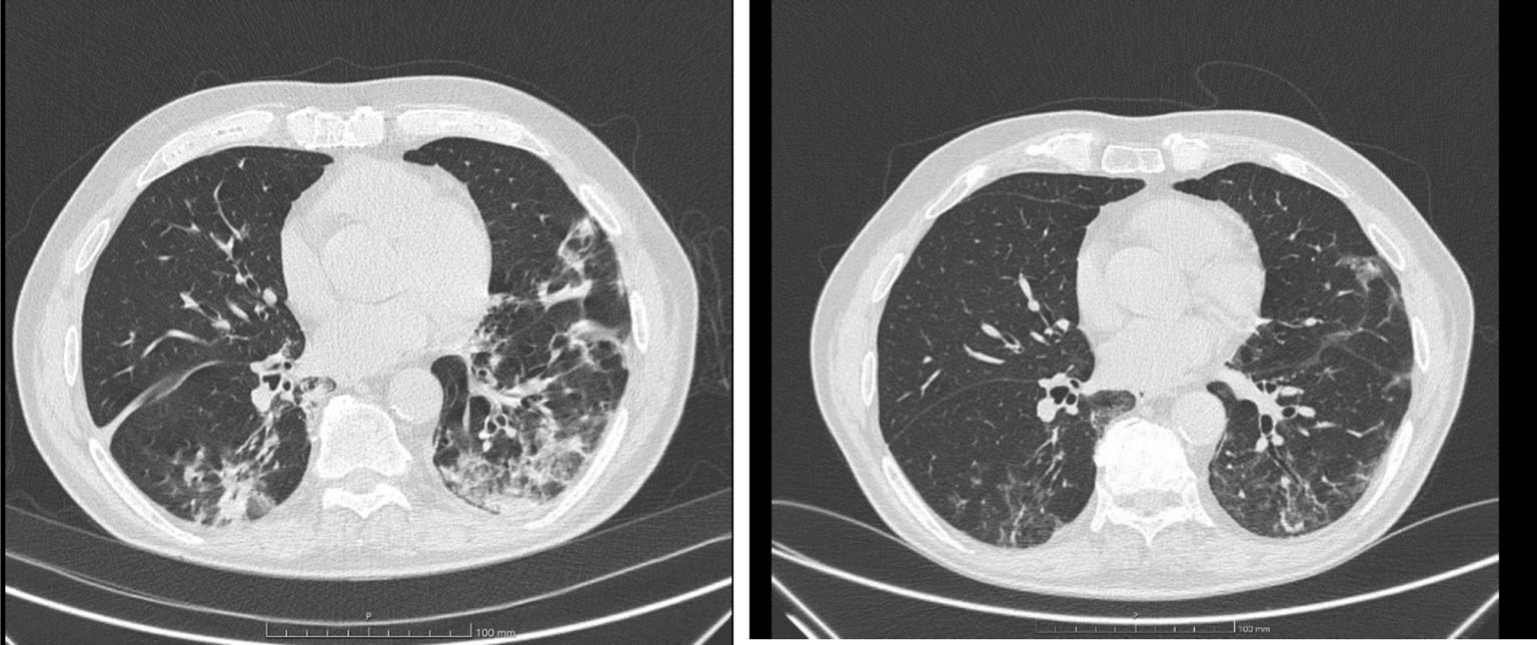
(a) Chest CT scan after 10 days of therapy; BAL positivity for fungal overinfection and A influenza did not show significant improvement (b) chest TC scan at 2 months follow‐up showing almost complete resolution of radiological abnormalities.
